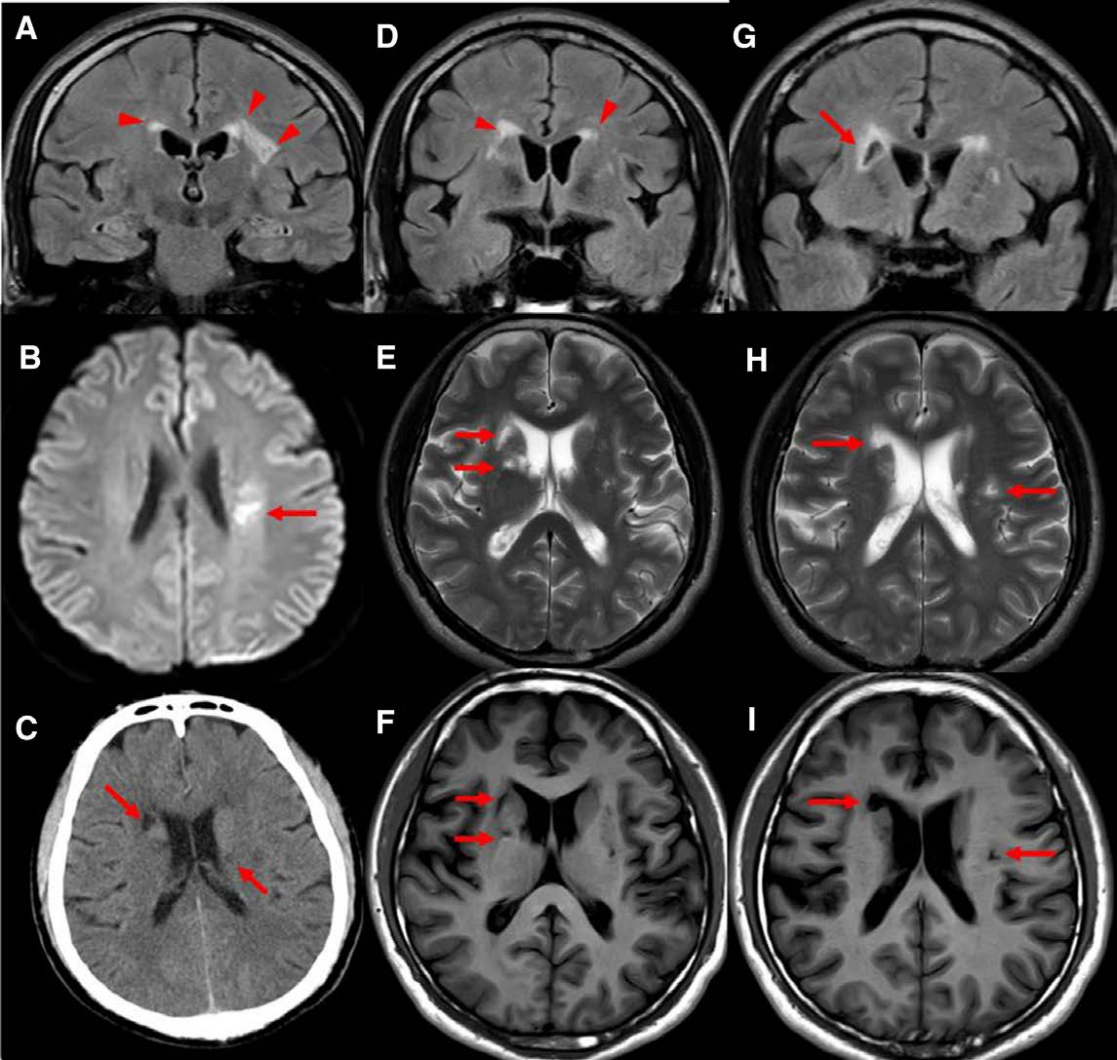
The CT scanning and MRI map during hospitalization. (A and D) white matter lesions in periventricle with hyperintensities on FLAIR (red arrow heads). (C) The cerebral infarctions on CT (red arrows). (B) Recent infarct in the left centrum semiovale on DWI (a red arrow); (E–I) Lacune of presumed vascular origin surrounding ventricle on T2WI, T1WI and FLAIR image (red arrows). CT = computed tomography, DWI = , FLAIR = fluid-attenuated inversion recovery, MRI = magnetic resonance imaging, T1WI = T1-weight, T2WI = T2-weight.
